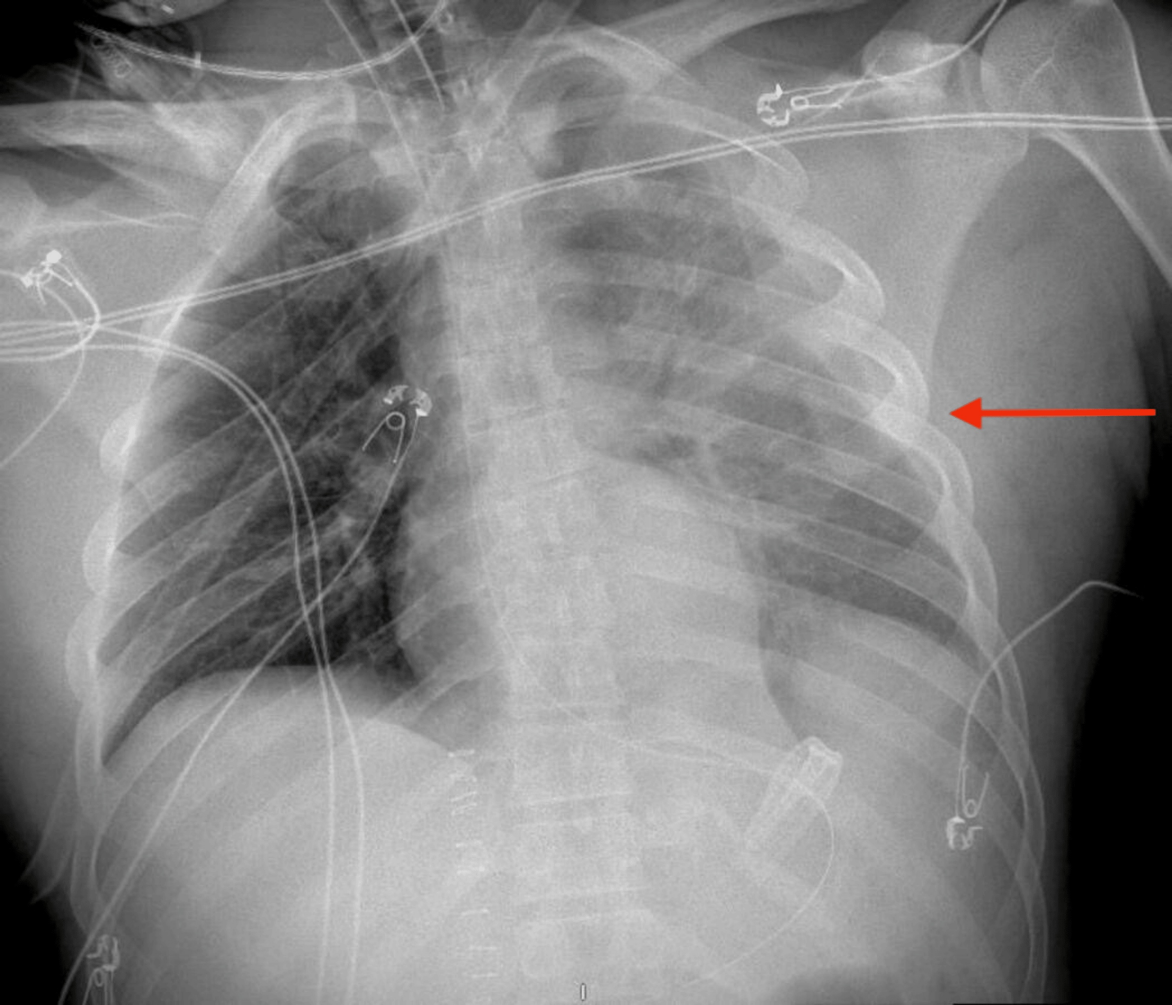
Chest X-ray showing left hemothorax (red arrow).
